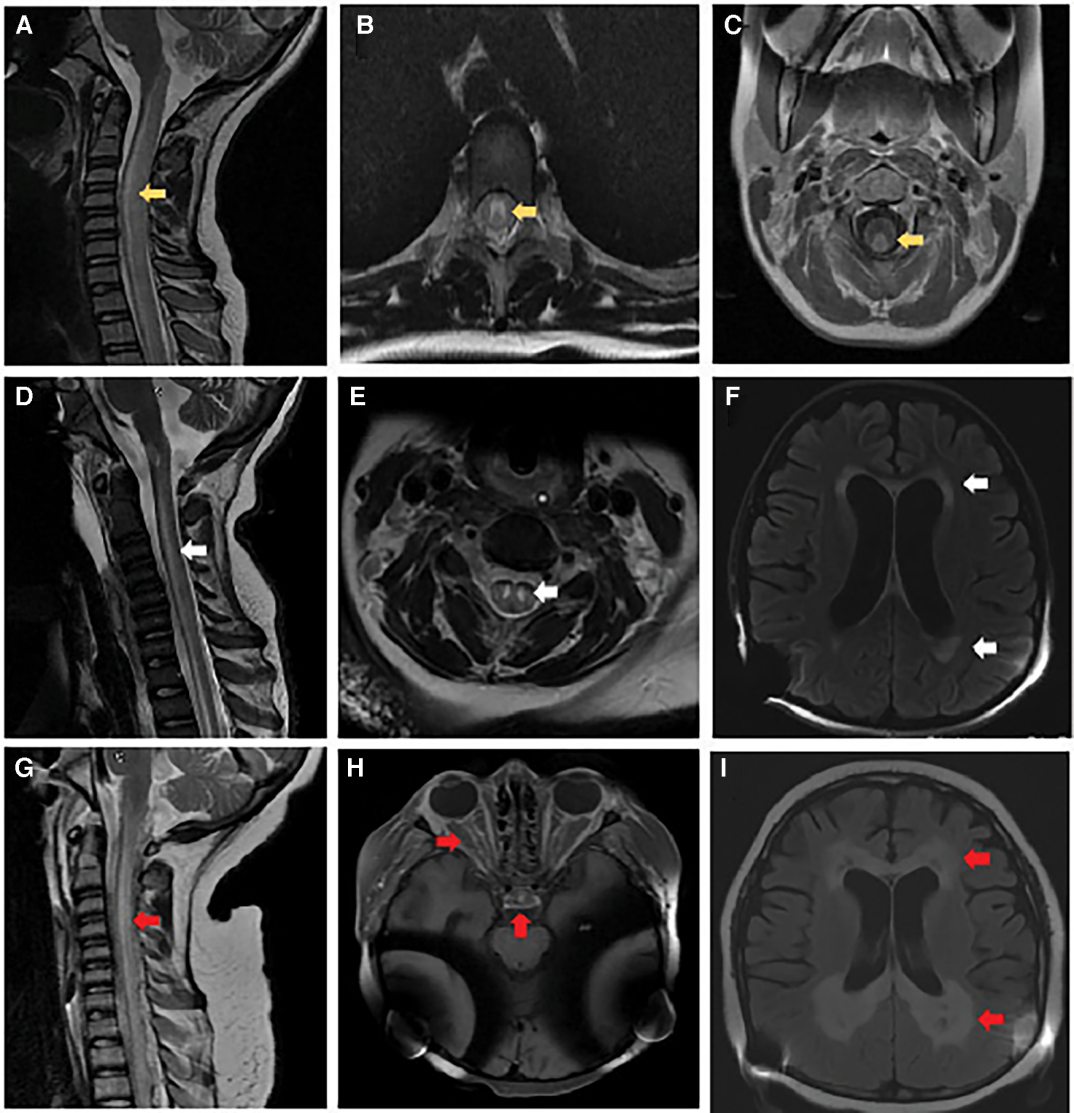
Brain and spinal cord imaging. At initial presentation: (A) MRI cervical spine sagittal T2-weighted image with hyperintense signal involving the central gray matter, dorsal and lateral tracts of the cervical and thoracic spinal cord (yellow arrow). (B) MRI thoracic spine axial T2-weighted image with hyperintense signal involving primarily gray matter (yellow arrow). (C) MRI cervical spine axial post-gadolinium T1-weighted image with contrast enhancement of the dorsal white matter tracts (yellow arrow). Follow-up at 6 months: (D) MRI cervical spine sagittal T2-weighted image with re-demonstration of central gray matter hyperintensity of the cervical and thoracic spinal cord with associated volume loss (white arrow). (E) MRI cervical spine axial T2-weighted image with increased hyperintense signal involving primarily gray matter and volume loss within the gray matter tracts (white arrow). (F) MRI brain axial T2-weighted FLAIR image with diffuse parenchymal volume loss and nearly symmetric bilateral periventricular and deep white matter signal abnormality in the frontal, parietal, and occipital lobes (white arrows). Obtained 2 weeks before death: (G) MRI cervical spine sagittal T2-weighted image with recurrent non-enhancing hyperintense signal involving primarily the dorsal central aspect of the spinal (red arrow). (H) MRI brain axial T1-weighted imaging post-gadolinium with contrast enhancement involving the optic chiasm, prechiasmatic optic nerves, and bilateral optic tracts within the suprasellar region (red arrows). (I) MRI brain axial T2-weighted FLAIR image with progressive bilateral periventricular white matter signal abnormality with central areas of white matter necrosis (red arrows).
